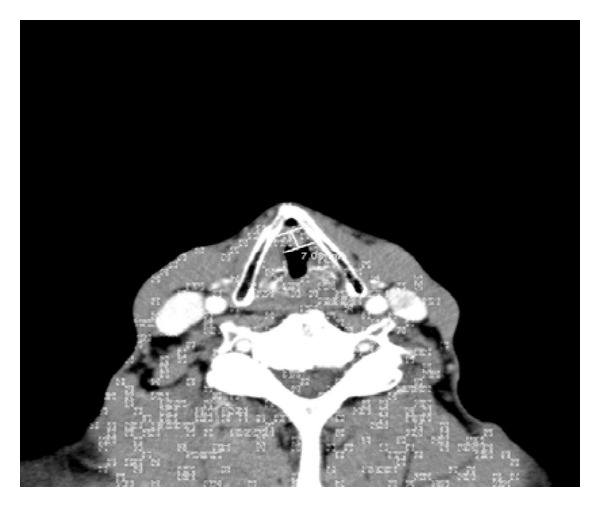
CT of the neck with contrast: There is 7 mm polypoid mass involving the anterior commissure of the vocal cords. The nasopharynx, and pharynx, peripharyngeal spaces appear unremarkable, as well as the parotid and submandibular salivary glands. There is no significant neck adenopathy noted.
